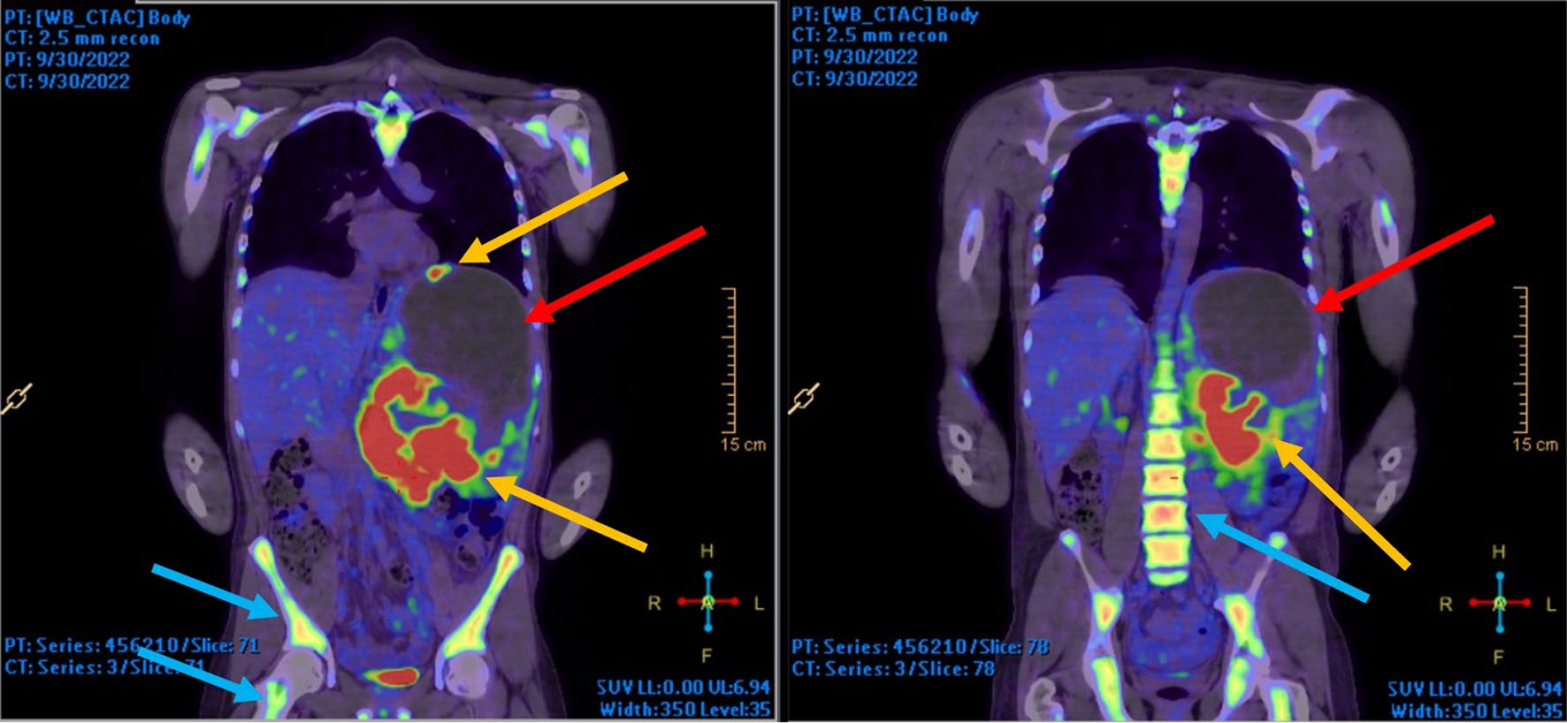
PET–CT images demonstrating tracer uptake in splenic periphery (orange arrows) and skeleton (blue arrows), as well as photopenic defects in the splenic core (red arrows)
